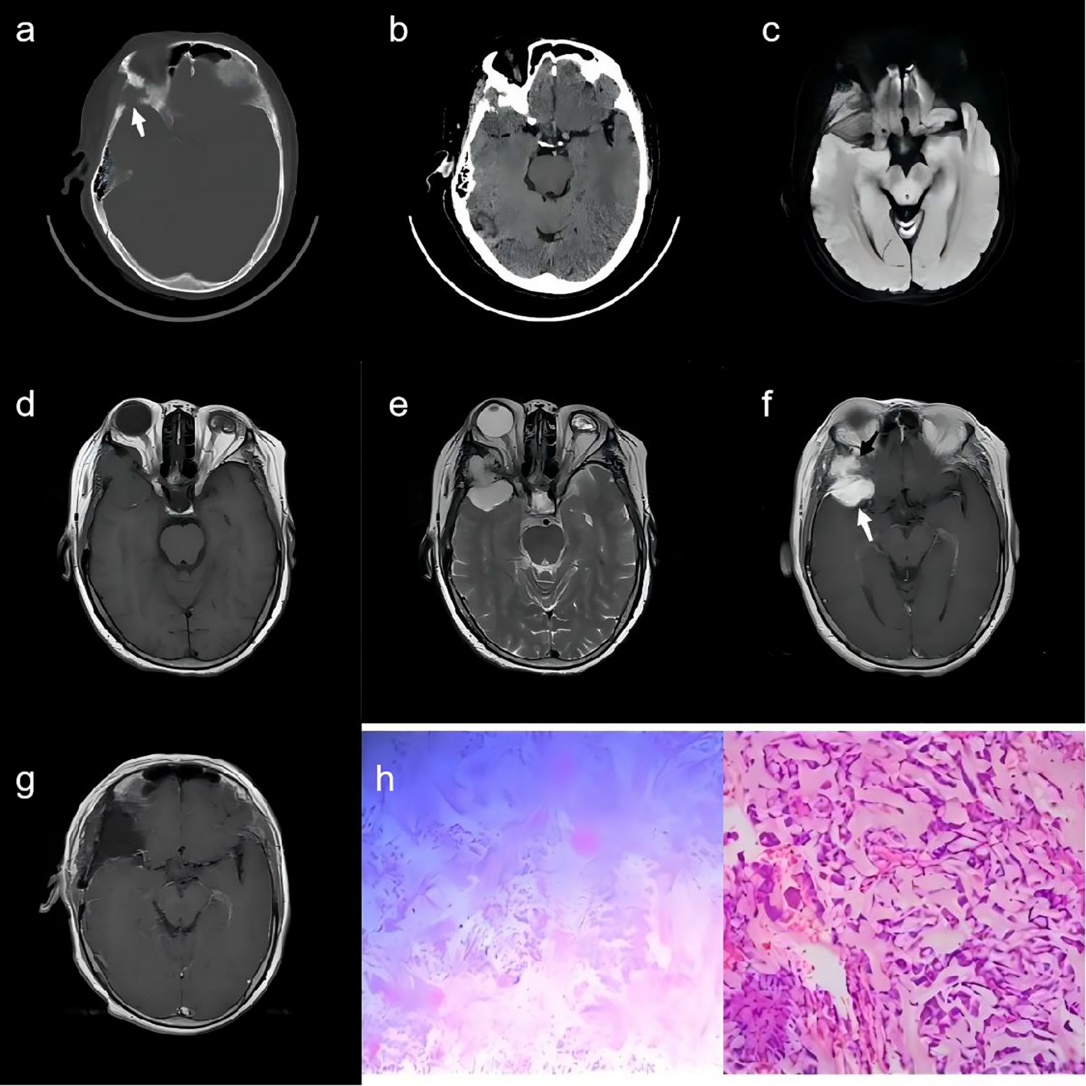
A 68-year-old woman. (A) A CT scan with bone window demonstrates the anterior part of the right temporal bone destruction (white arrow). (B) A Brain CT scan shows a rounded-like soft-tissue density mass in the temporal region. (C–E) MR images depict a dumbbell-shaped intradiploic mass that was iso-hypo signal intensity on T1WI (D) and diffusion sequence (C), and hyperintensity on T2WI (E). (F) The lesion shows intense and heterogeneous enhancement in two parts (white arrow and black arrow, respectively) on contrast-enhanced T1WI. (G) The post-operative contrast-enhanced T1WI revealed no significant enhancement in the lesion region. (H) Pathological examination revealed chordoid meningioma (CNS WHO grade II) with eosinophilic vacuolated tumor cells on a myxoid background.
